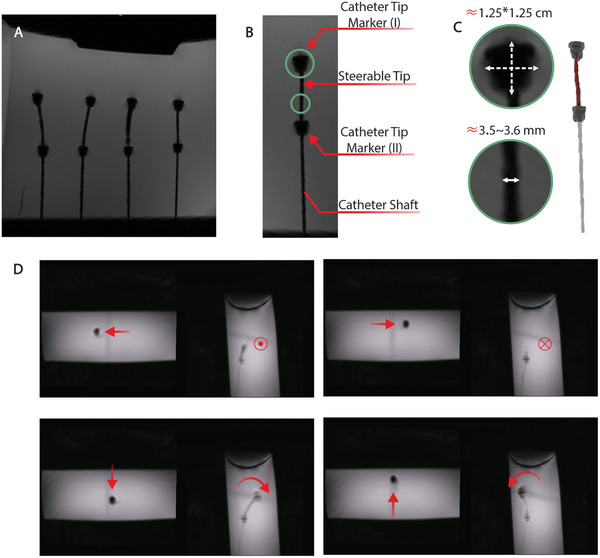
In vitro MRI test for steerable catheter. A) Imaging artifacts of four steerable catheters acquired using a 3D Flash sequence at 3 Tesla. B) Labeling of the artifacts and inset of the catheter tip marker artifacts (upper) and catheter shaft artifacts (lower) with dimensions. C) Reconstructed 3D model of the steerable catheter from the acquired scan. D) Real‐time imaging in the transverse and sagittal planes as the catheter is steered in all four directions.
